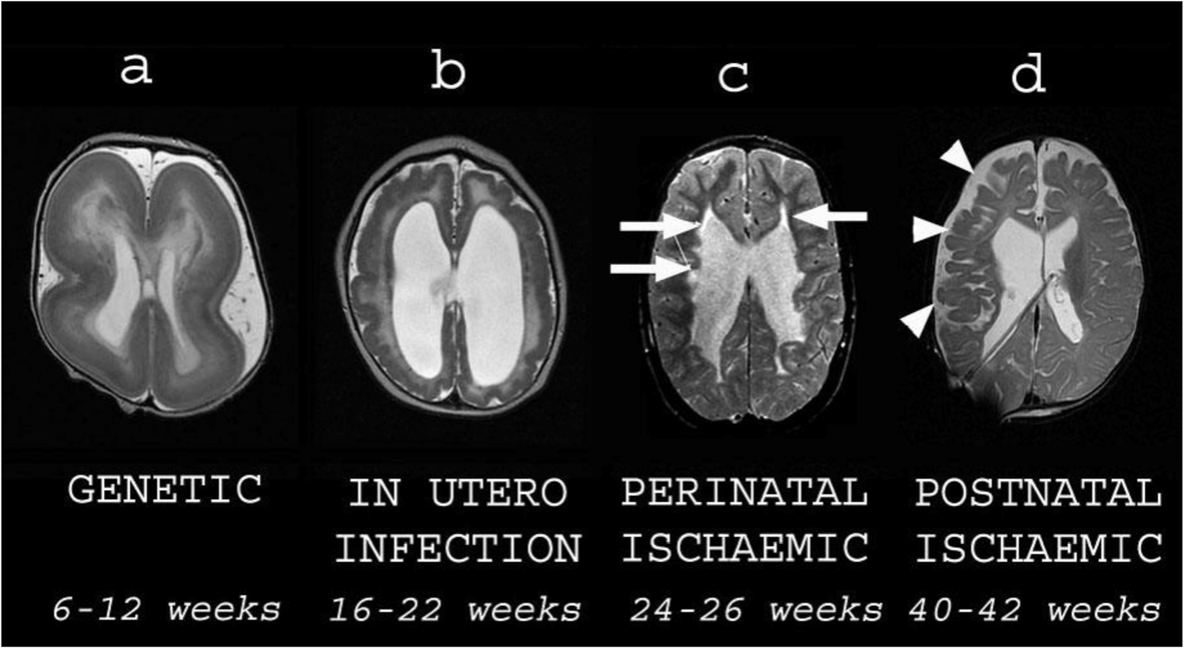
Examples of different types of structural brain abnormalities in cerebral palsy All images are axial T2-weighted MRI scans. Each image is subtitled by its presumed aetiology and timing during gestation. a is a child with lissencephaly showing cortical thickening and agyria. b is a child with congenital cytomegalovirus infection showing an overfolded cortex (polymicrogyria), thin white matter and dilated lateral ventricles. c is an ex premature child showing cystic white matter injury (arrows) consistent with periventricular leukomalacia. d is a child who suffered a haemorrhagic stroke in the newborn period. There is cortical and white matter loss in the right frontal and parietal lobes (arrowheads) consistent with previous ischaemia.
